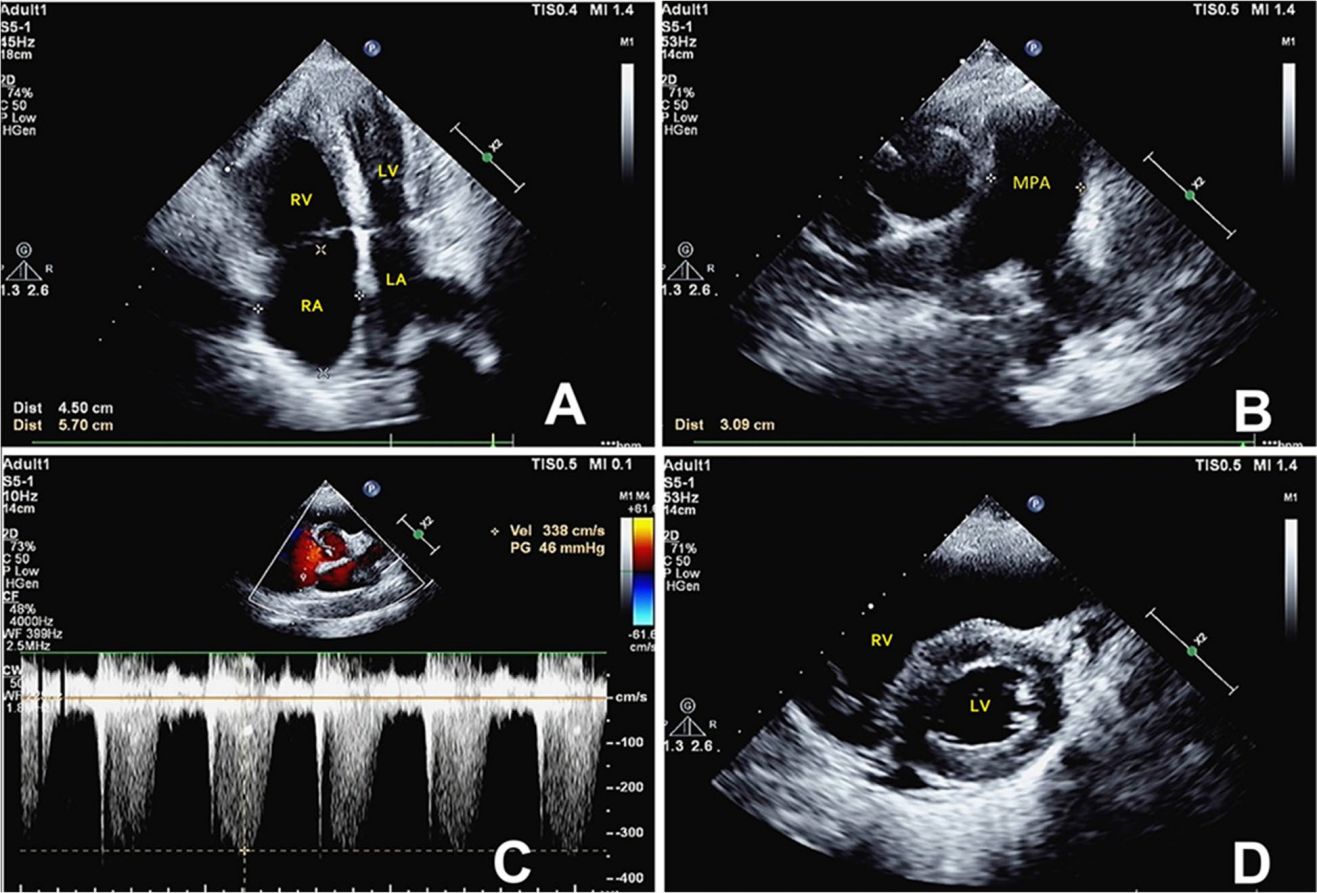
Pre-treatment echocardiography (A-D). Apical four-chamber view showing enlargement of right atrium (RA) and right ventricle (RV) (A); Short-axis view of the heart showing dilation of the main pulmonary artery (MPA) and its branches (B); Peak tricuspid regurgitation velocity by continuous Doppler, peak right ventricle–to–right atrial systolic pressure gradient is 51 mmHg (C); Left ventricle short-axial view showing symmetric slight thickening of the left ventricle (LV); LV end-diastolic wall thickness is 13 mm (D)
